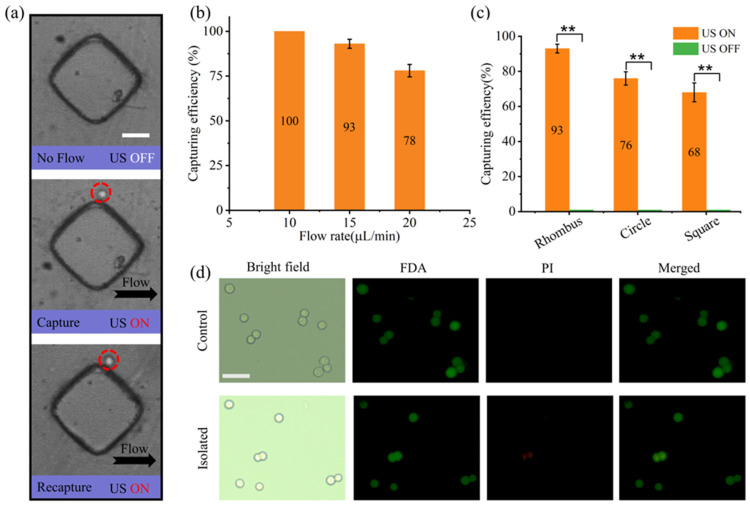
Flexibility, efficiency and biocompatibility of cancer cell capture by acoustic streaming. (a) The ability to flexibly capture the same cancer cells at different micropillar positions in the capture area by simply starting and stopping the ultrasound device. (b) Capture efficiencies of rhombus AMPA chips at flow rates of 10, 15, and 20 µL/min. (c) Impact of three micropillar shapes in AMPA chip on the capture efficiency of cancer cells in whole blood samples. (d) Bright-field and untreated cancer cell samples (upper panel) and fluorescence images of acoustically separated (lower panel). Error bars represent standard deviation, and all experiments were repeated (n = 3). All scale bar: 50 µm. *p* < 0.01 ().
